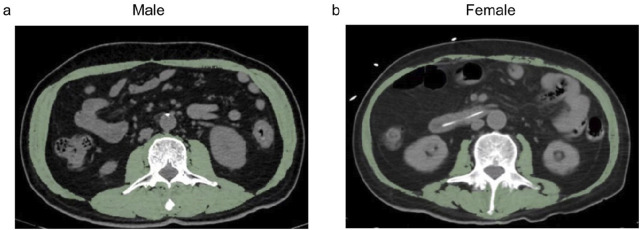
Representative images of L3 axial CT slices of skeletal muscle distribution in initial-dialysis patients. Both skeletal muscle area and density were measured in (a) male patients and (b) female patients.
